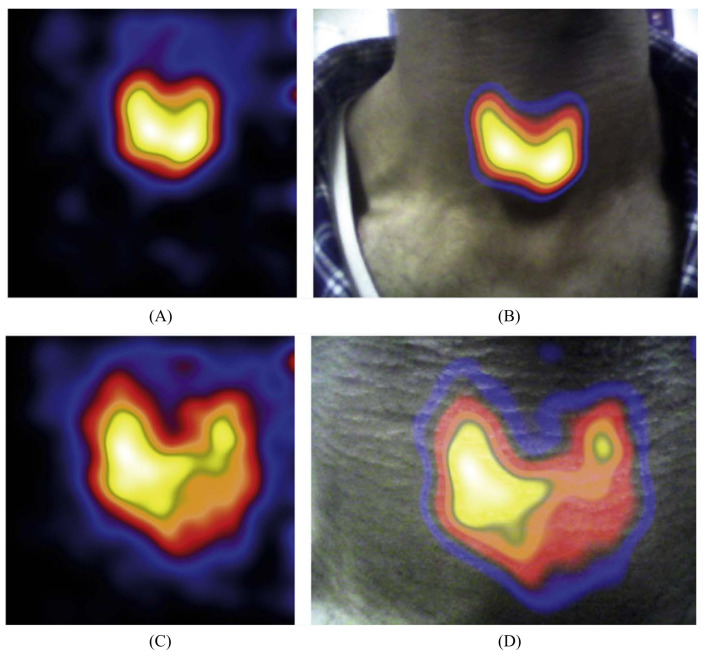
Non-surgical gamma and gamma–optical thyroid scintigraphy images acquired using the HCGC. Reproduced from Bugby et al. [23]. (A) Gamma-only thyroid image acquired at ~17 cm distance; (B) Combined gamma–optical image of the gamma-distribution shown in (A); (C) Gamma-only thyroid image acquired at ~8 cm distance; (D) Combined gamma–optical image of the gamma-distribution shown in (C).
